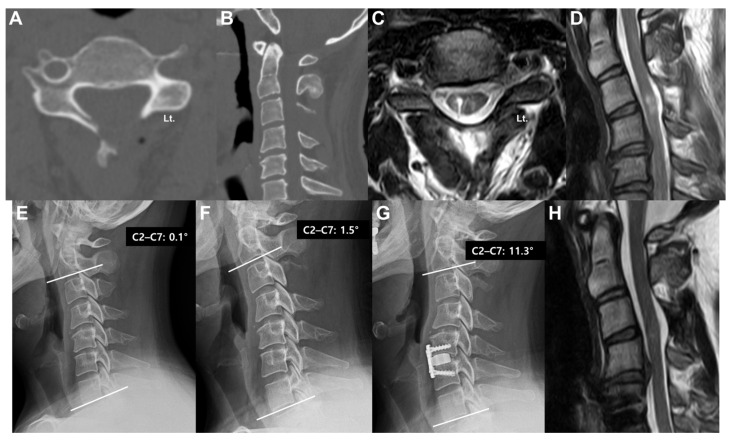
(A,B) Postoperative axial and sagittal CT. (C,D) Postoperative axial and sagittal T2-weighted MRI showing resolved cord compression with residual high signal intensity (D). (E–G) Preoperative, immediate postoperative, and final follow-up at 18 months, lateral radiographs (C2–C7 Cobb angles: 0.1°, 1.5°, and 11.3°). The patient underwent C5–C6 anterior cervical diskectomy and fusion (ACDF) for an unrelated disc herniation at 2 months postoperatively (visible in (G)). (H) Sagittal T2-weighted MRI at 18 months postoperatively, demonstrating partial regression of the cord signal change seen on D and no evidence of recurrence.
